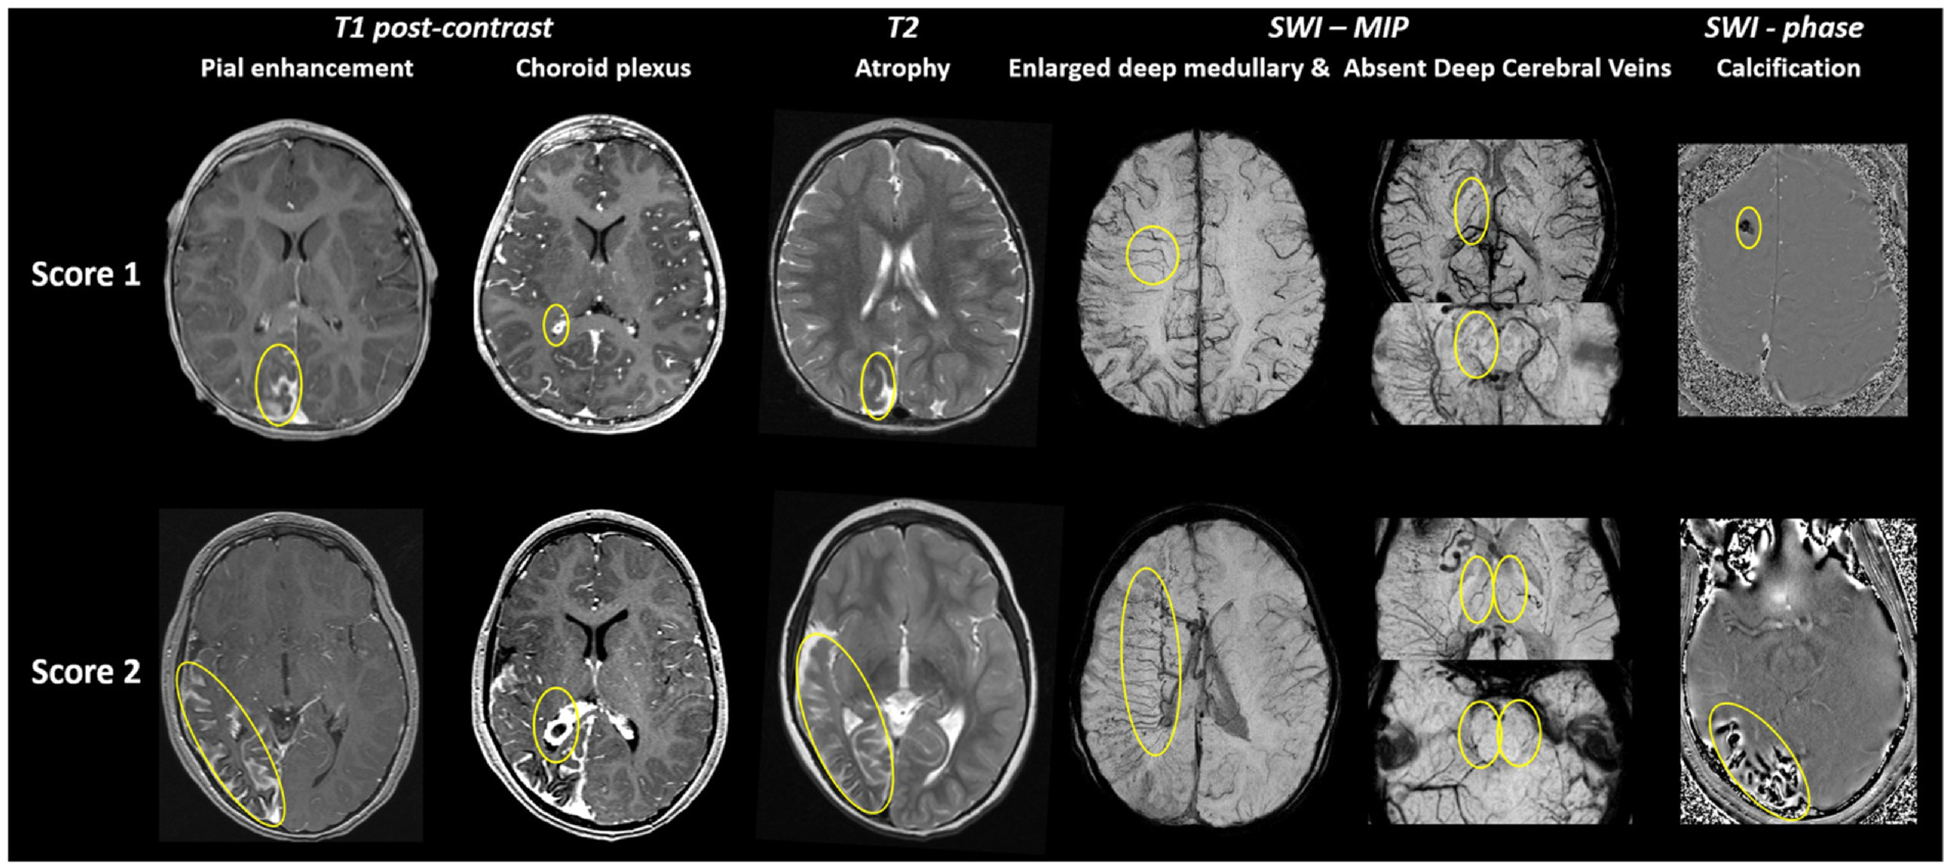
Relevant MRI sequences used for MRI scoring, showing venous vascular and parenchymal abnormalities, with examples of scores 1 and 2 for each feature (abnormalities are circled). Pial enhancement: sublobar (score 1), extensive lobar (score 2); choroid plexus: mild asymmetry (score 1) vs. robust asymmetry (score 2); atrophy: sublobar (score 1) vs. extensive lobar (score 2); enlarged deep medullary veins: sublobar (score 1) vs. extensive lobar (score 2); absent deep cerebral veins: unilateral absence of the internal cerebral vein (upper panel) and the basal vein of Rosenthal (lower panel) vs. bilateral absence of the same veins (score 2); calcification: small sublobar (score 1) vs. extensive lobar (score 2).
